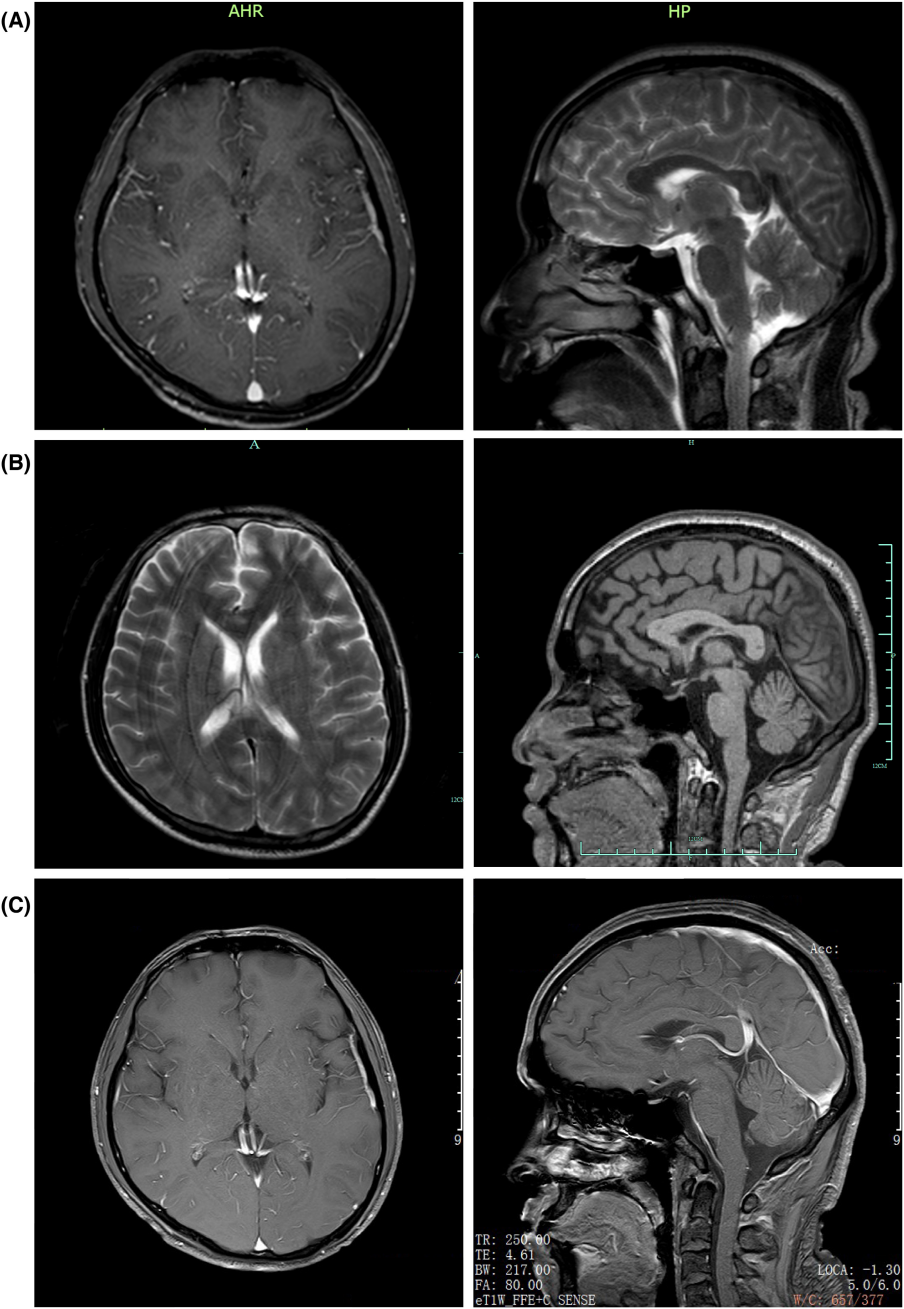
Brain MRI images of the patient. (A) Brain MRI on June 23, 2022. (B) Brain MRI on July 16, 2022. (C) Brain MRI on July 23, 2022.
